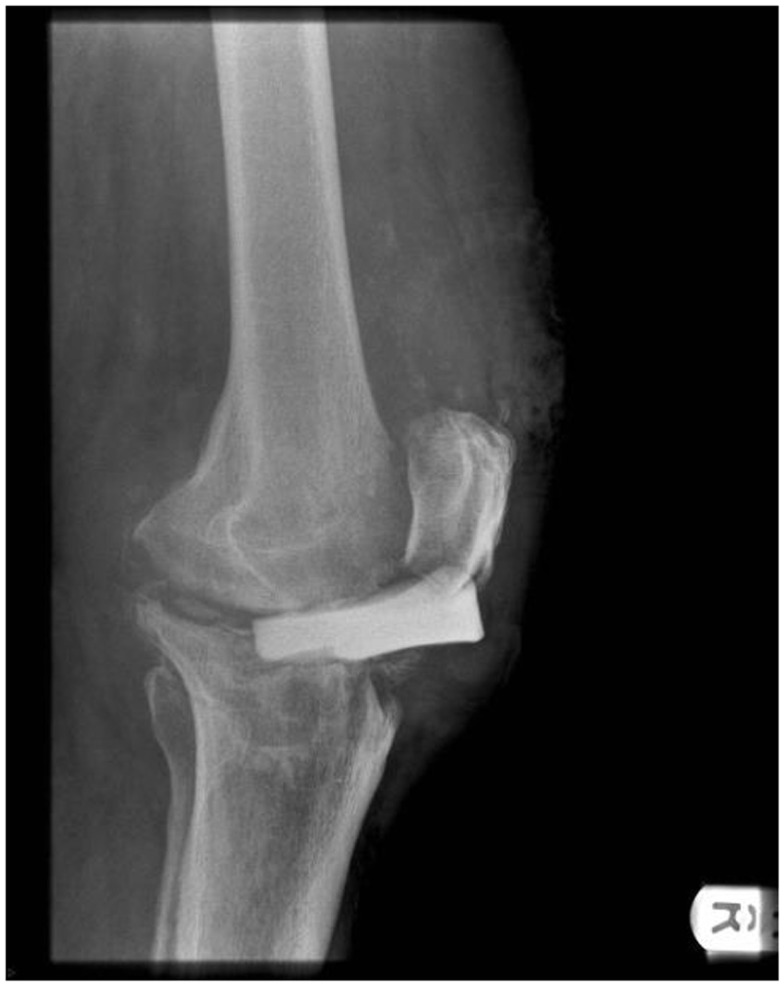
Complete patella tendon rupture in Case 1 demonstrated by the lateral radiographs of the right knee showing significant anterior displacement of the static cement spacer block. Note that the block was not keyed in.
